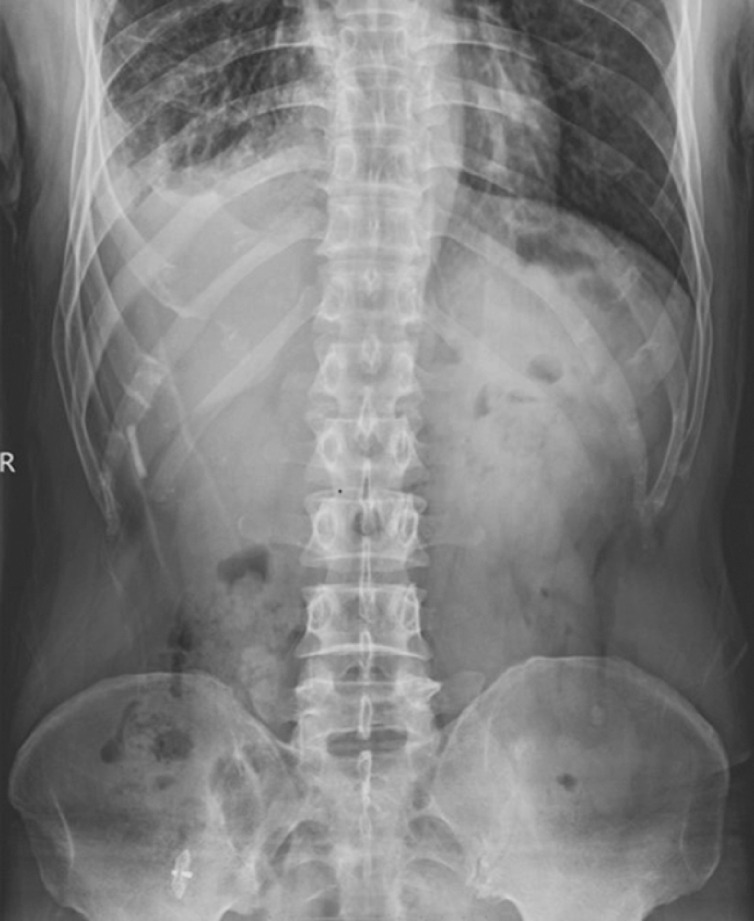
Digital radiography examination revealed that the occluder had fallen off into the colon.
